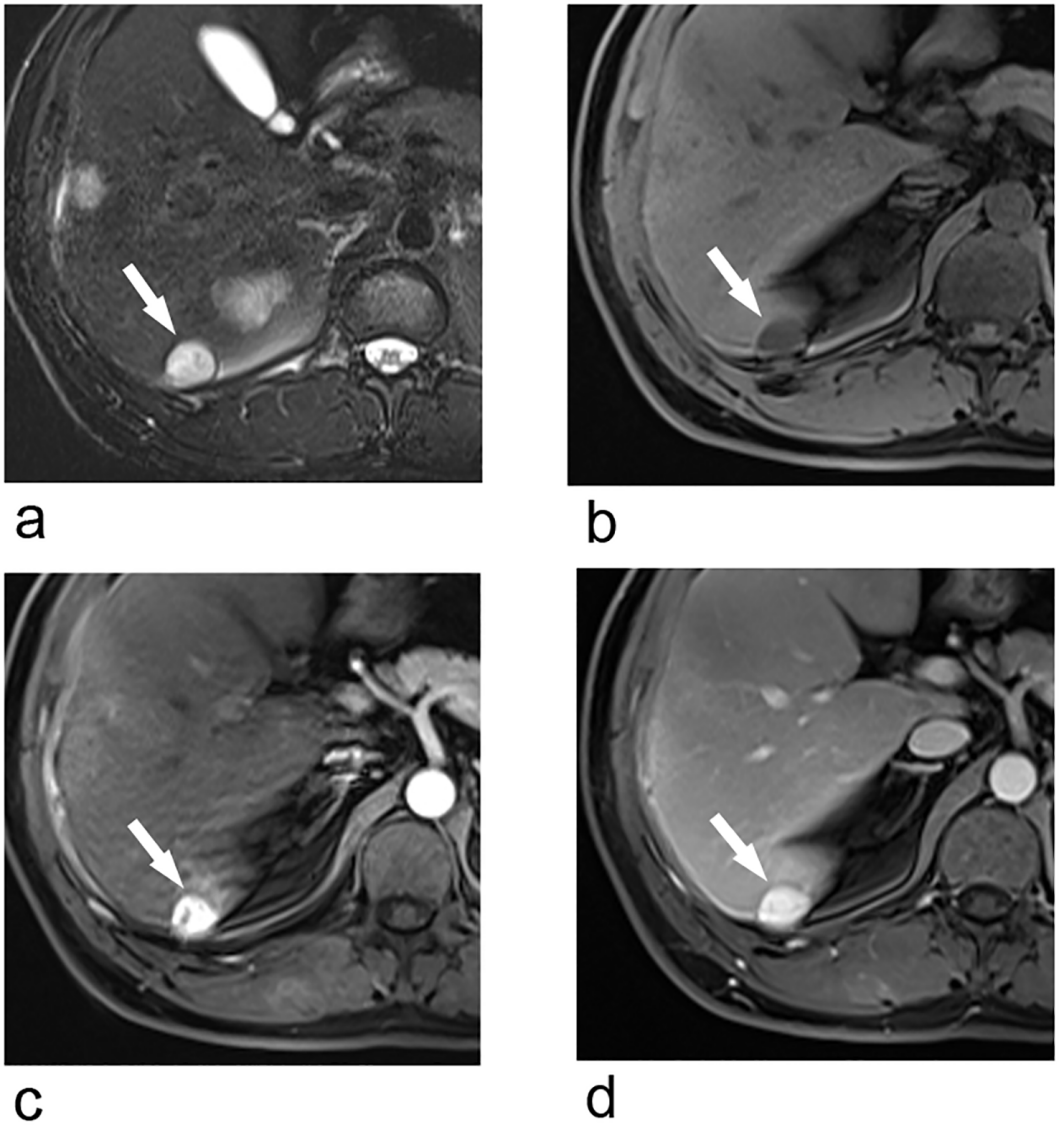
Transverse breath-hold turbo spin-echo T2-weighted image (repetition time msec/echo time msec, 3000/104) (a) and fat-suppressed T1-weighted volume interpolated body examination (VIBE) image (repetition time msec/echo time msec, 3.92/1.39) (b) shows a well-circumscribed liver nodule. Transverse arterial phase (c) and portal venous phase (d) show marked enhancement of the nodule.
